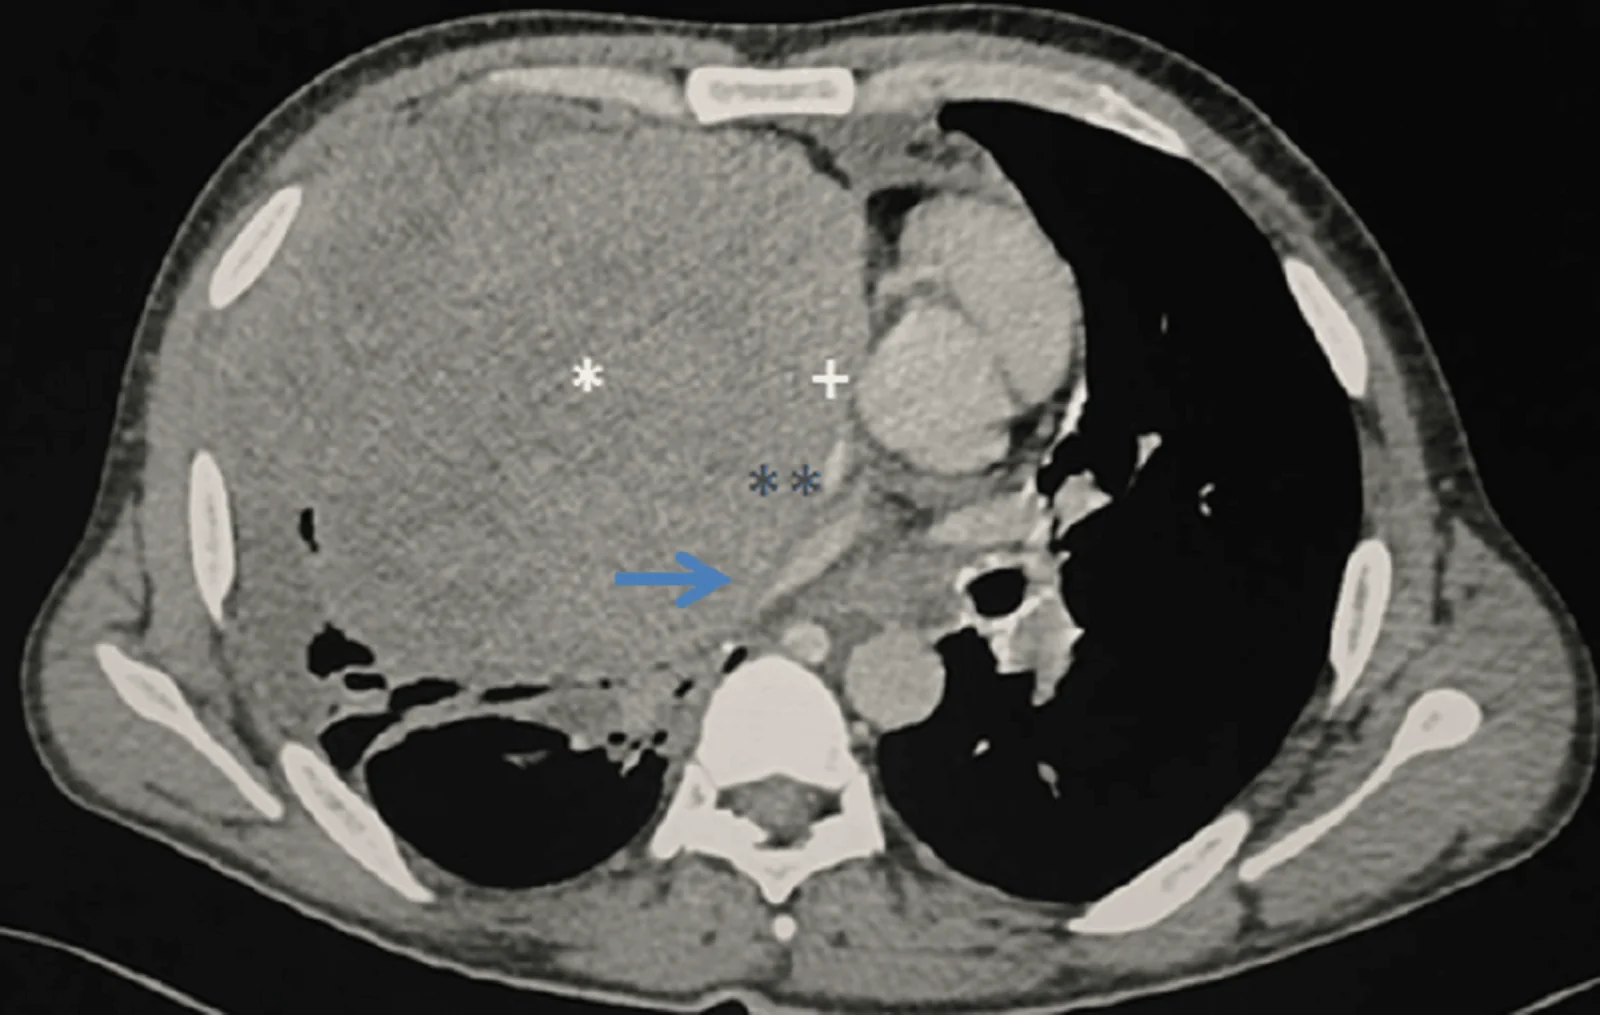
Axial contrast-enhanced chest computed tomography (CT) scan showing a large mediastinal mass (*) with its mass effect in the cardiac structures: The superior vena cava (white plus sign, +), the right main pulmonary artery (blue double asterisk,), and the right atrium (blue arrow, →)
